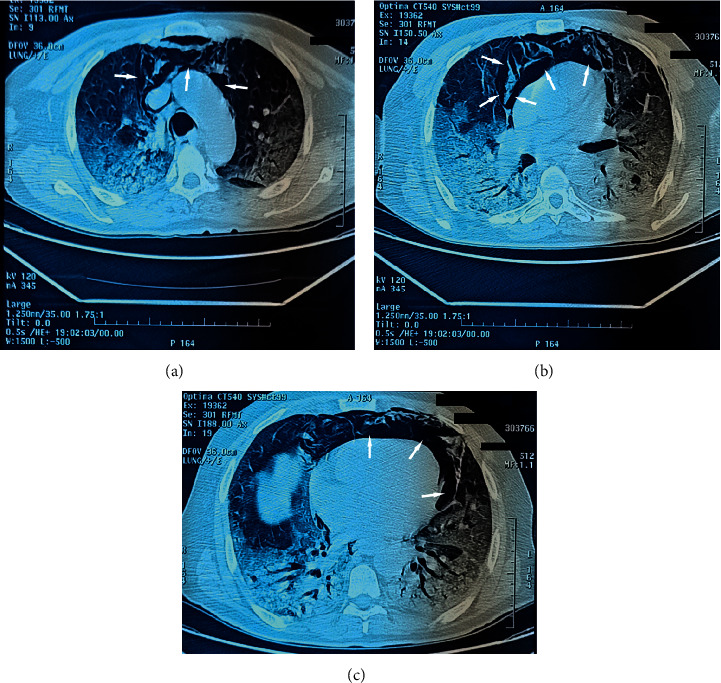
(a, b, c) Chest CT scan showing ground-glass opacities with crazy paving of mixed topography, bilateral to lower predominance with a degree of critical involvement >75% classified CORADS 5 and a large abundance of spontaneous pneumomediastinum.
